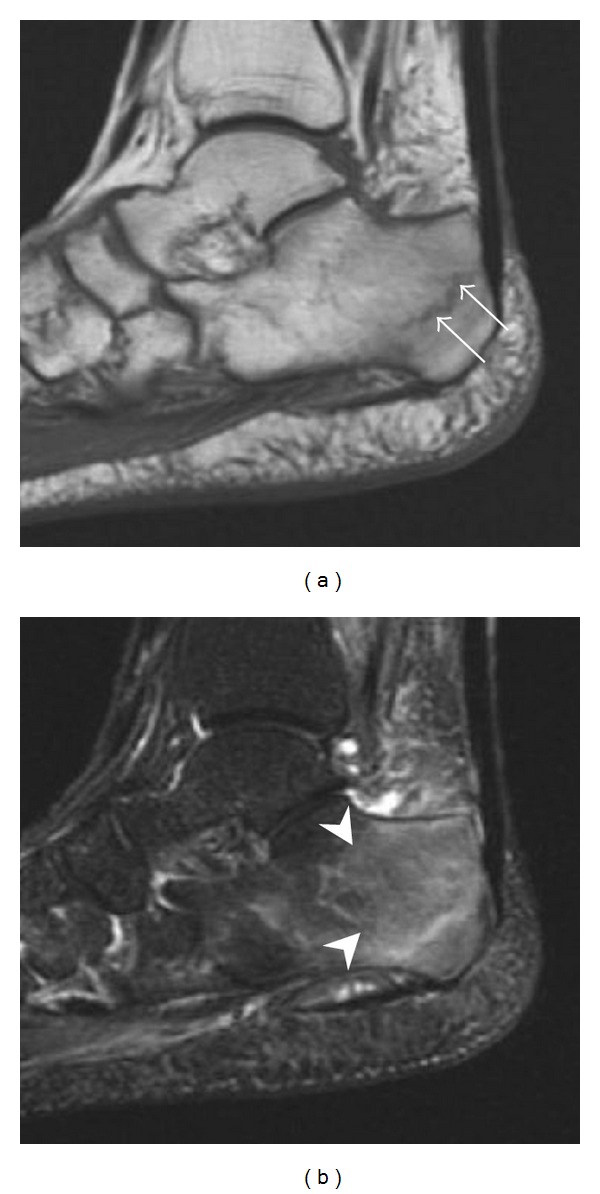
Calcaneal fatigue fracture in a 30-year-old male runner. Radiographs were normal (not shown). (a) Sagittal T1-weighted and (b) short tau inversion recovery images show a linear hypointensity (arrows) of calcaneal tuberosity within diffuse bone marrow edema, which appears as an ill-defined area of hyperintensity on a fluid sensitive pulse sequence (arrowheads).
